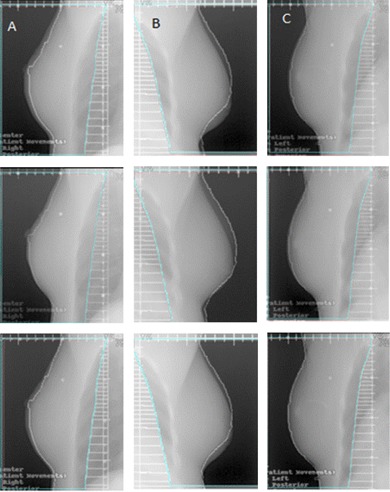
Digitally reconstructed radiograph and MV film comparison for three different patients (Columns A, B, and C). The interpatient variation is shown across the rows, while the intrapatient variation is shown down each column. Each tick‐mark on the axis represents 1 cm.
